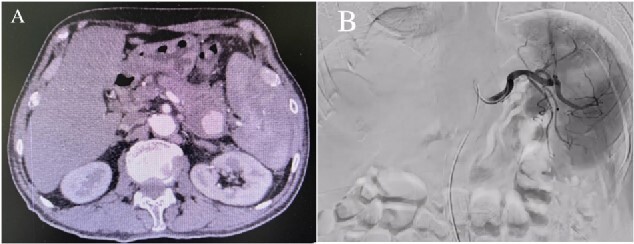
(A) Abdominal CT scan showed the presence of a SAP. (B) Celiac angiography confirmed that the main trunk of the splenic artery showed contrast agent overflow and the formation of a pseudoaneurysm of the splenic artery.
